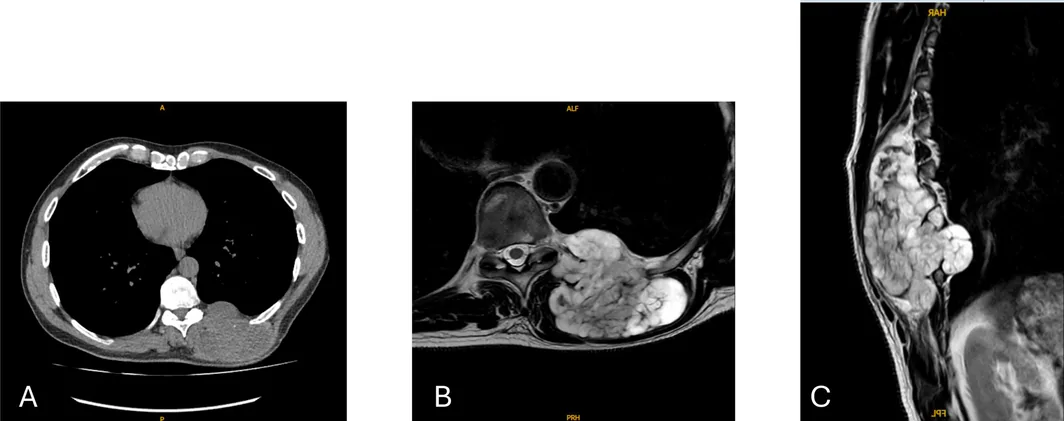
(A) Axial thoracic CT showing a left paravertebral mass involving ribs 9–10 with endothoracic extension. (B) Axial thoracic MRI demonstrating the relationship between the tumor, pleural cavity, and paraspinal muscles. (C) Sagittal thoracic MRI showing craniocaudal extension and contact with vertebral elements.
